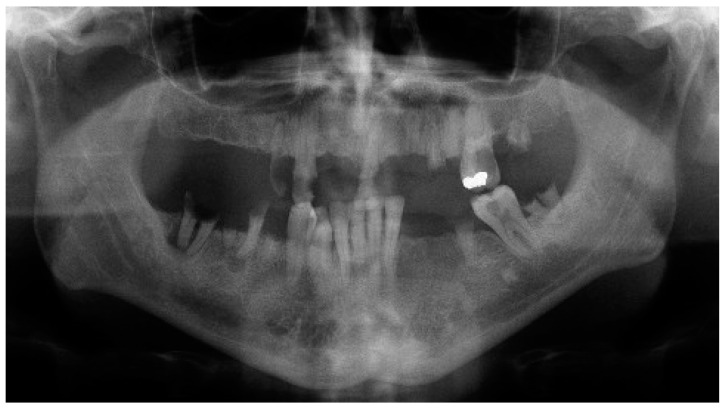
Preoperative panoramic radiograph of patient 1 included in the present research.
